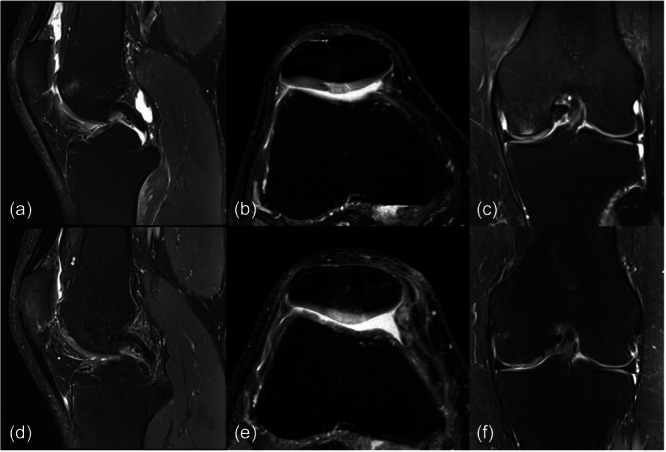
Preoperative MRI (a–c) of chondral defects in the trochlea (a), retropatellar (b) and at medial condyle (c). Corresponding postoperative MRI after the minced cartilage procedure (d: 14 months, e: 12 months, f: 24 months).
